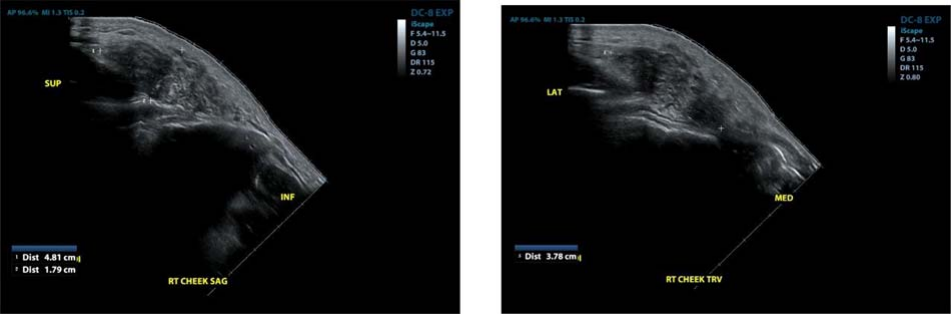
Ultrasound examination demonstrating a heterogenous lesion measuring 4.8 × 3.8 × 1.8 cm mass.
